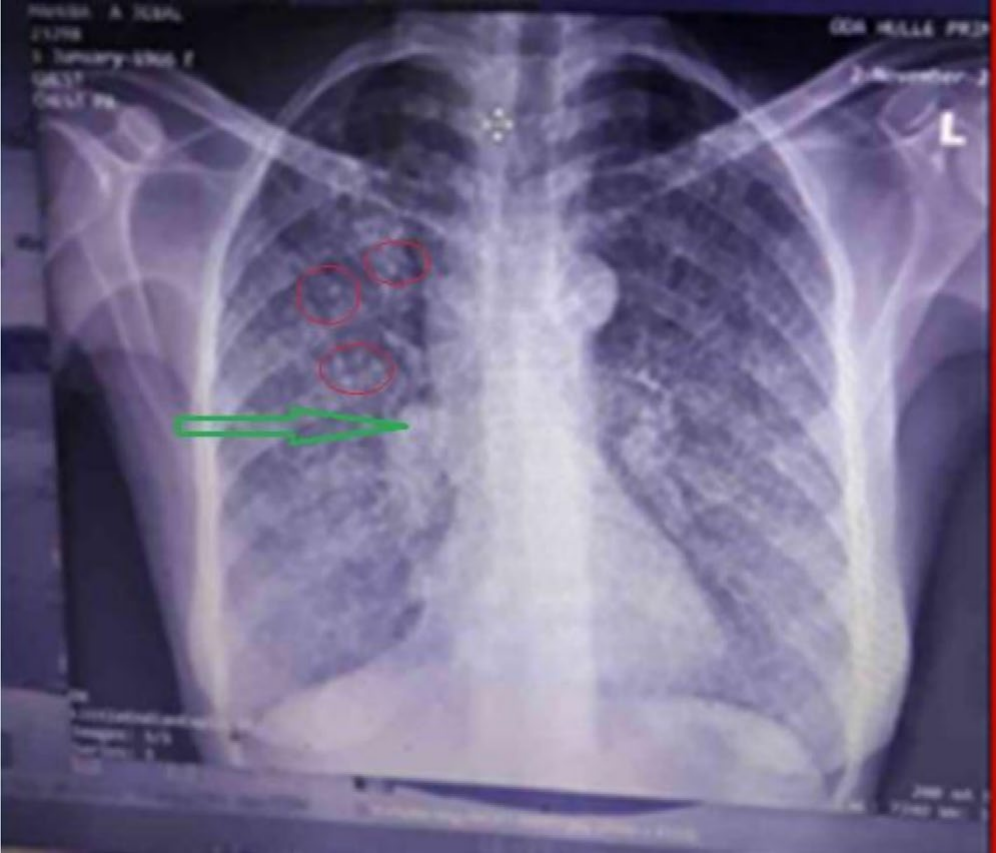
An anteroposterior chest radiograph demonstrates bilateral, diffuse miliary nodules (1–2 mm) and right hilar lymphadenopathy. Red circle indicates miliary nodules and green arrow indicate an enlarged right hilar lymph node, findings characteristic of miliary tuberculosis.
